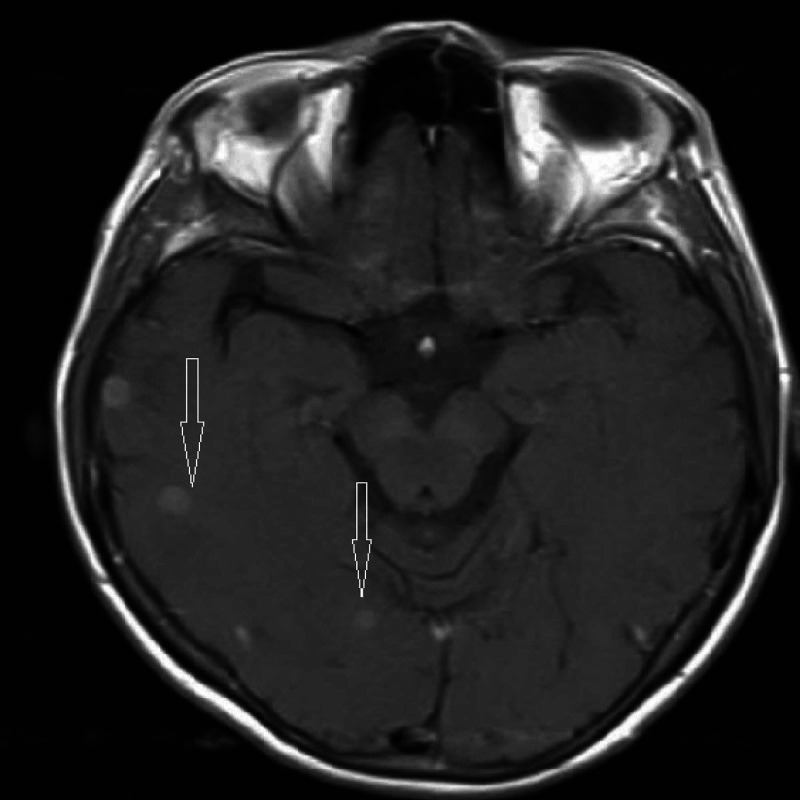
MRI-Brain MRI-brain showing brain metastasis (arrows)
